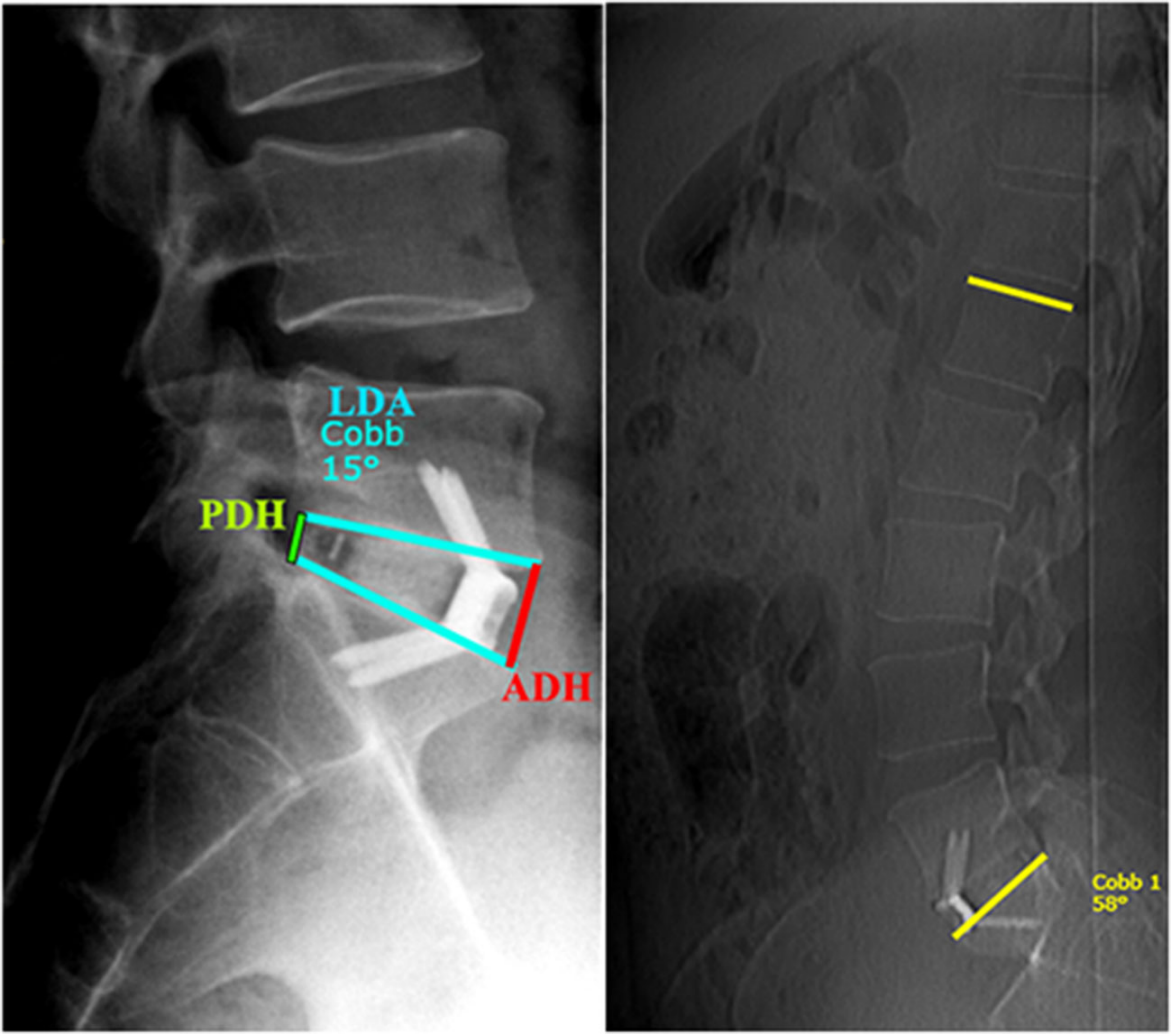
Preoperative and postoperative changes in neural foramen dimensions—foramen height (FH), foramen width (FW) and area of the foramen (FA) calculated on CT scans with P-P technique
